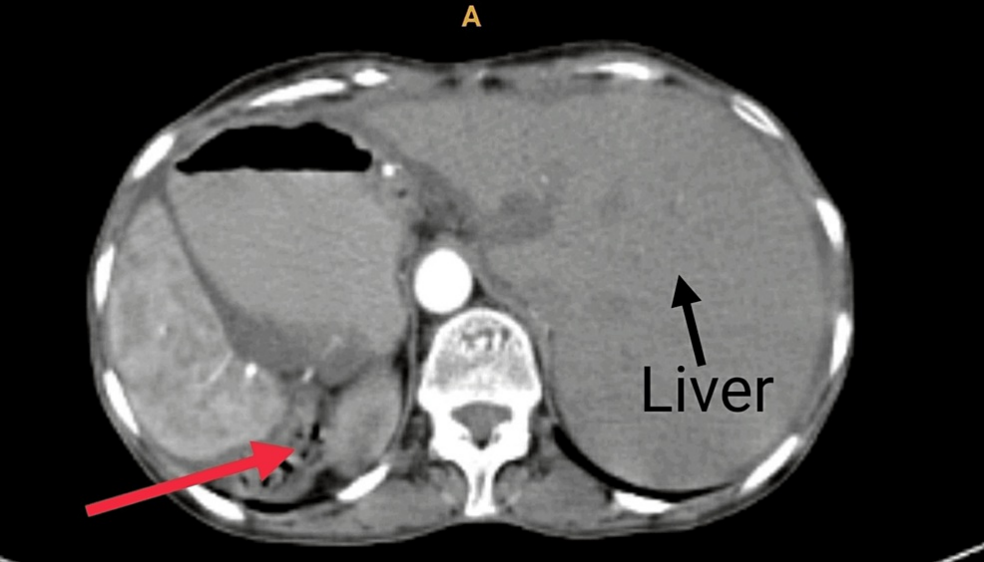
Contrast-enhanced computed tomography of the abdomen. The red arrow shows a thickening of the pyloric wall suggestive of neoplasm, and the black arrow shows the liver on the left side of the abdomen. There is no evidence of metastatic lesions in the liver or involvement of para-aortic lymph nodes.
